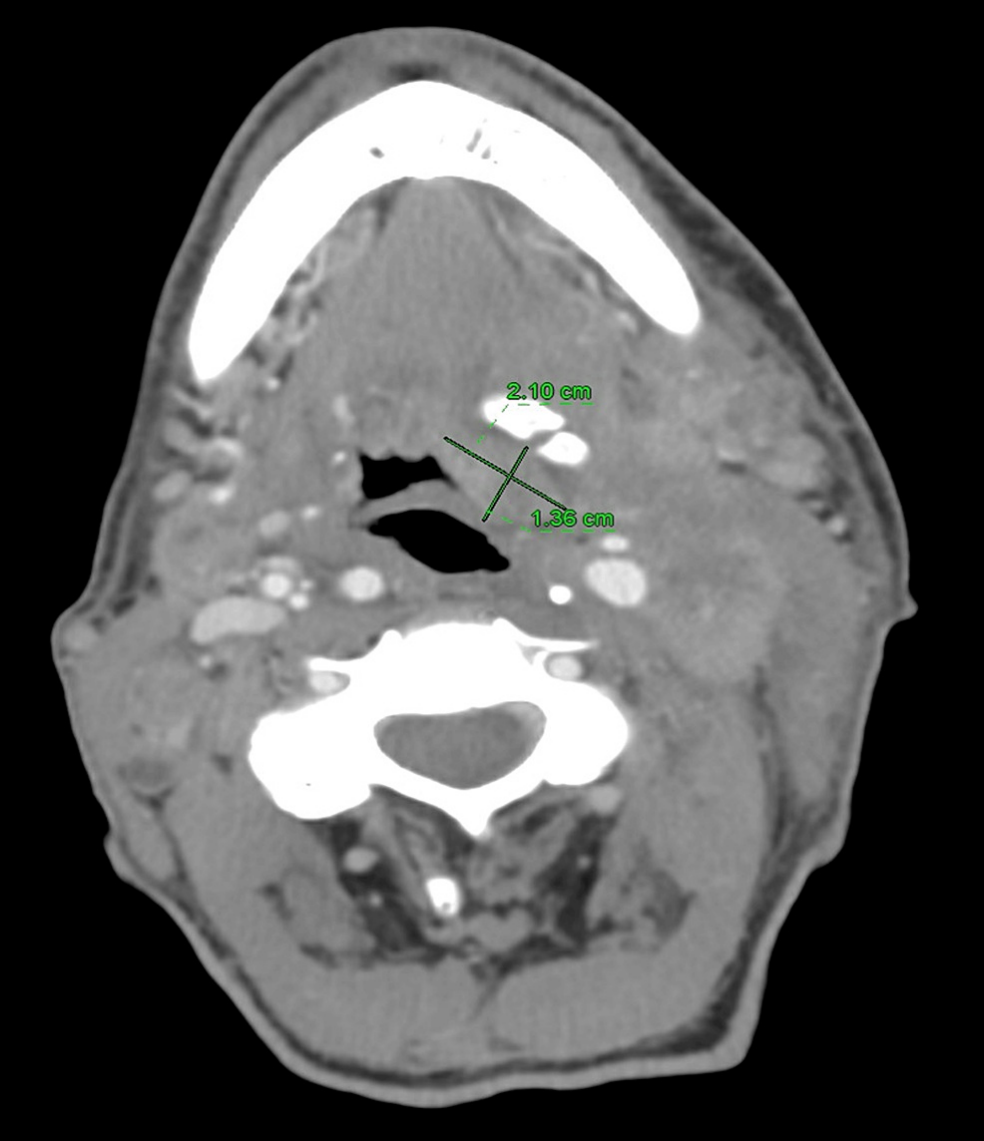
CT soft tissue neck with IV contrast Imaging demonstrates an ill-defined soft tissue mass within the left hypopharynx and extending into the left pre-epiglottic vallecula. This is indistinct. However, it measures approximately 5 cm x 2.2 cm x 1.7 cm (green). Diffuse bulky necrotic cervical lymphadenopathy is noted, most consistent with squamous cell carcinoma.
